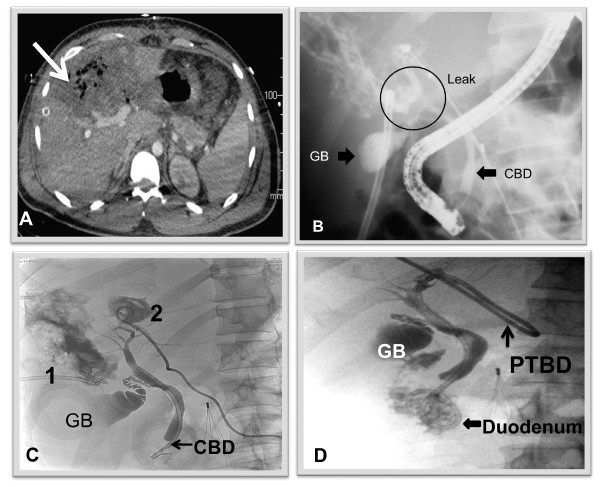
A 25 year old male suffered a trans-abdominal GSW with grade 4 liver injury, duodenal and small bowel injuries. On PID 12 intrahepatic abscess was found on CT scan (A, arrow). A bile leak developed following percutaneous drainage. An ERCP (B) was performed and showed a leak from both right and left hepatic ducts (GB - gallbladder, CBD - common bile duct, leak from both ducts is encircled). Following image (C) showed well-drained intrahepatic bilomas (percutaneous drains 1 and 2). PTBD was inserted instead of a percutaneous drainage (D). It was removed 4 months later when no contrast leak was found and the patient tolerated closure of the external part of the PTBD.
